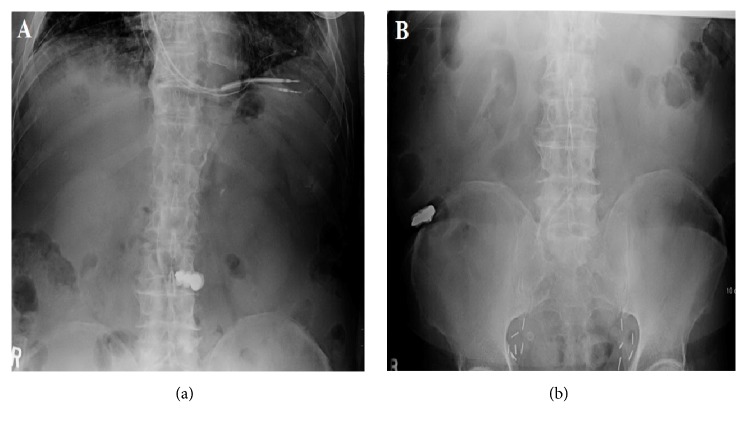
(a) The first abdominal radiograph demonstrating the dental plate in the mid abdomen. (b) The second abdominal radiography demonstrating the dental plate in the right lower quadrant and likely in the cecum.
